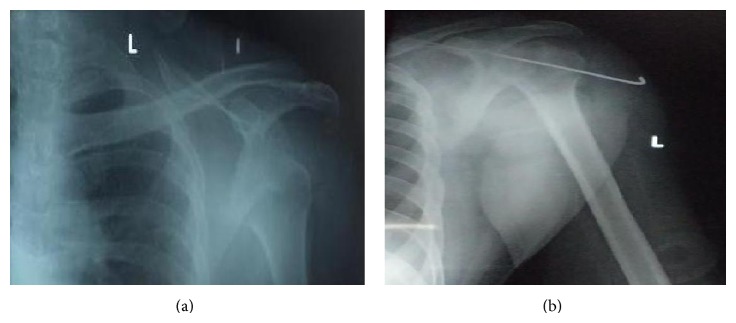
Radiographs of left anterior dislocation of shoulder (preoperative and postoperative).
